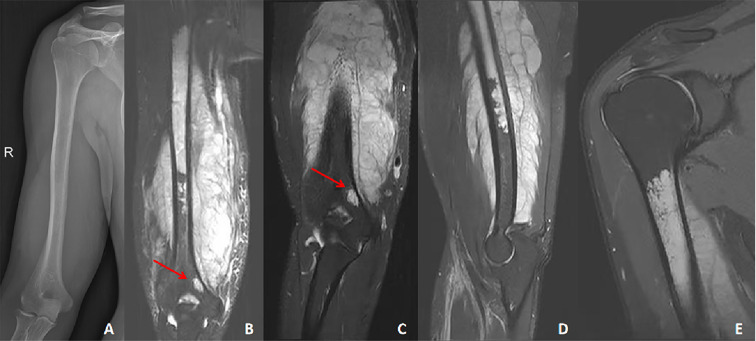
A 24-year-old patient with chondroblastic osteosarcoma of the humerus. (A) Pre-treatment radiography revealed normal bone mineralization of the humerus, with no evidence of osseous abnormality; however, a soft-tissue mass was observed in the mid- and distal shaft. (B) Pre-treatment MRI demonstrated a large tumor occupying the medullary cavity of the humeral shaft and extending into the surrounding soft tissues. A skip lesion was also identified at the humeral condyle (red arrow). (C, D) Post-treatment MRI showed marked reduction in tumor volume with well-defined margins, resolution of peritumoral edema, and persistence of a skip metastasis at the distal humeral condyle (red arrow). (E) Post-treatment MRI confirmed no tumor involvement of the humeral head.
